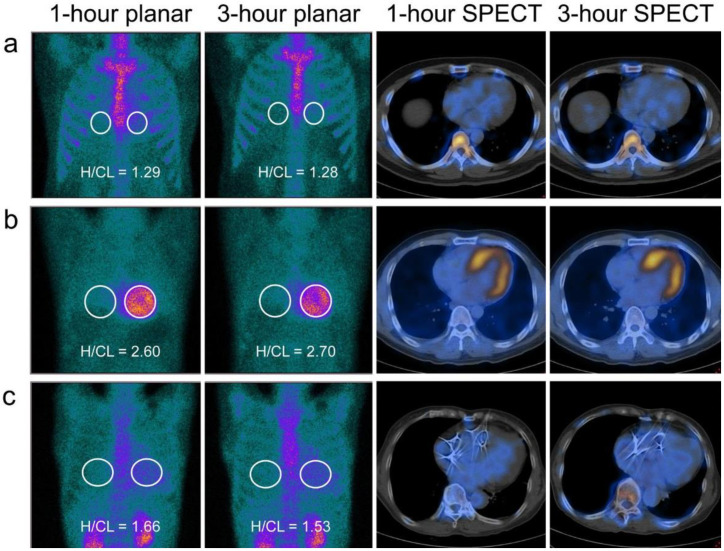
^99m^Tc-PYP planar and SPECT/CT scans obtained at 1 and 3 h in typical cases. White ellipse /circle of region of interest (ROI) was drawn over the precordium on anterior planar images, and mirrored to the contralateral chest for correcting the uptake in ribs and lung background. (a) The patient had negative planar studies at both 1 and 3 h based upon semi-quantitative visual grading scale relative to ribs (VGSr), visual grading scale relative to the upper segment of the sternum (VGSs), and heart-to-contralateral (H/CL) ratio approaches, respectively. 1- or 3-h SPECT/CT imaging allows identification of radiotracer activity in the left ventricular cavity other than the myocardium. (b) Despite the lack of heart failure symptoms, this patient had positive planar studies at both 1 and 3 h based upon semi-quantitative VGSr (both Grade 3), VGSs (both Grade 3) and H/CL ratio (2.60 and 2.70, respectively) approaches. Both 1- and 3-h SPECT/CT images showed diffuse ^99m^Tc-PYP uptake in the myocardium. As the immunoglobulin light chain test was negative and Asp38Asn mutation was detected, a definite diagnosis of hereditary ATTR-CA was achieved without processing an invasive endomyocardial biopsy. (c) Based upon semi-quantitative VGSr (both Grade 3) and H/CL ratio (1.66 and 1.53, respectively) approaches at 1 and 3 h, as well as 1-h VGSs (Grade 2) approach, the patient was considered as having positive planar studies. However, this “false positive” interpretation was refuted by Grade 1 referring to 3-h VGSs, with concordant to no radiotracer myocardial uptake on 1- and 3- hour SPECT/CT images.
